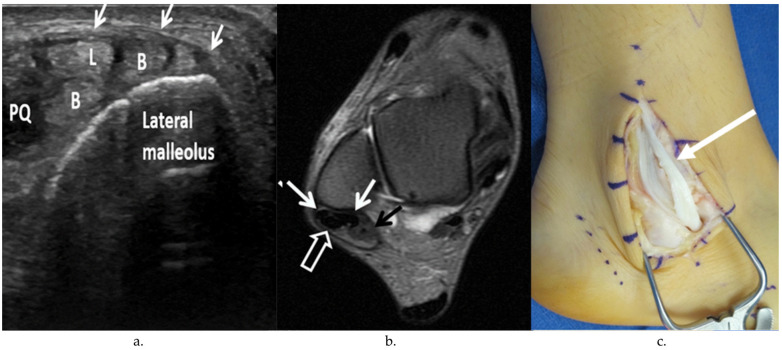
US, MRI, and intraoperative photos of peroneal tendon tears. (a) Transverse (short axis) grayscale US image of the right ankle in the retromalleolar region shows a longitudinal split tear of the peroneus brevis tendon with 2 hemi-tendons (B), intact peroneus longus tendon (L), and accessory peroneus quartus (PQ) under the superior peroneal retinaculum (SPR) (arrows). (b) Axial T2-weighted MR image with fat saturation of the right ankle shows a longitudinal split tear of the peroneus brevis tendon (white arrows) in the retromalleolar region. The peroneus longus tendon (open arrow) is intact. Note peroneus quartus at the posteromedial aspect of the peroneal tendons (black arrow). (c) Intraoperative image of the lateral aspect of the right ankle showing a longitudinal split tear of the peroneus brevis (black arrow).
